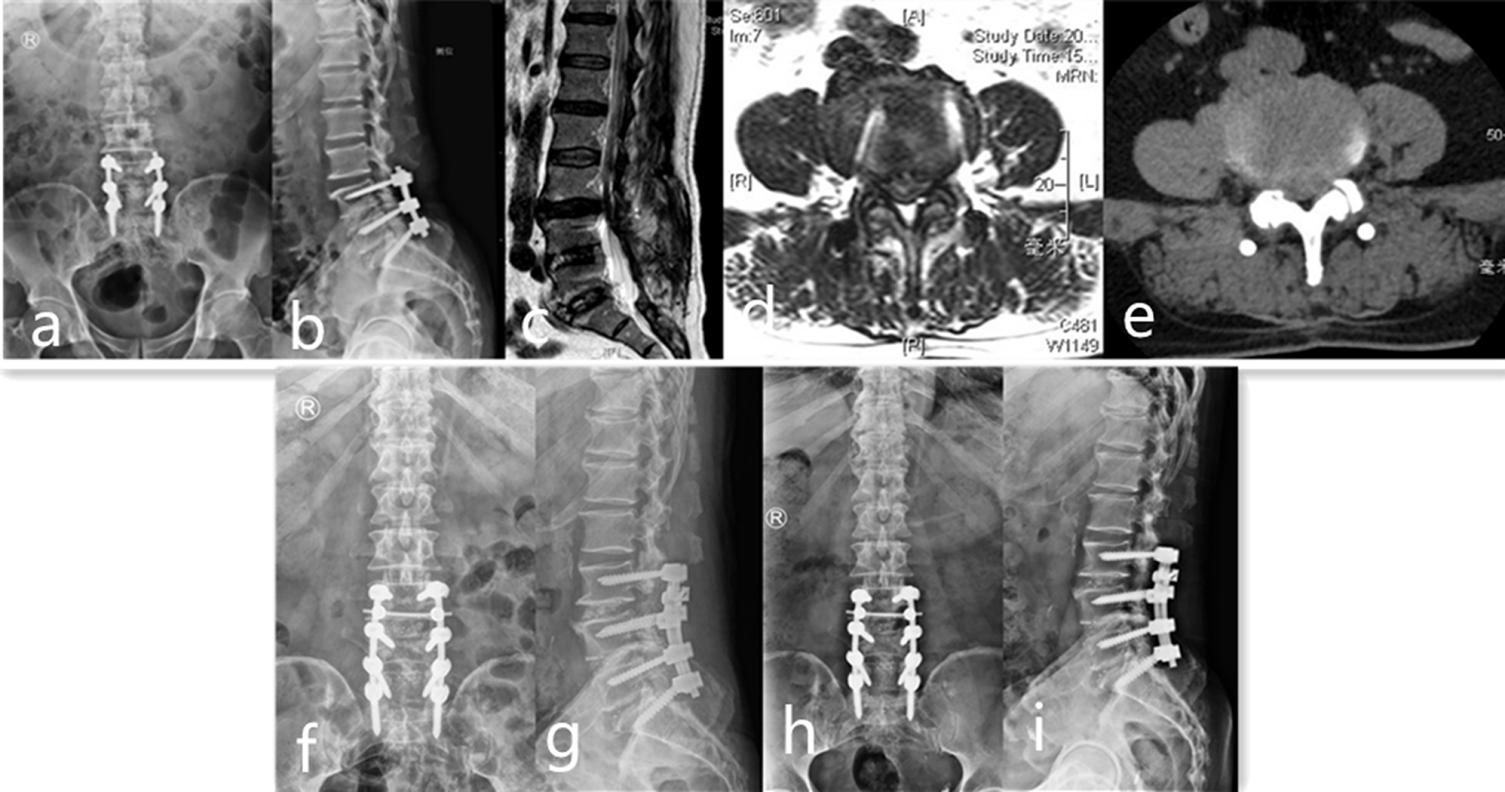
A 65-year-old female was diagnosed as symptomatic ASD at the L3/4 level. He was performed with posterior decompression and internal fixation at L4-S1 due to disc herniation 2 years ago. a, b Preoperative X-ray showed posterior fixation with an intervertebral cage at L4/5 and mild posterior displacement at L3/4. c, d, e, f Preoperative MRI and CT showed disc herniation at L3/4, which compressed dura sac and L4 nerve root. f, g Postoperative X-ray showed posterior decompression and fixation surgery was performed at L3/4 and longer rods were used to connect the ASD level with the primary surgical sites. h, i Postoperative X-ray at 1-year follow-up showed good results were achieved and no instrumentation breakage and displacement were found
